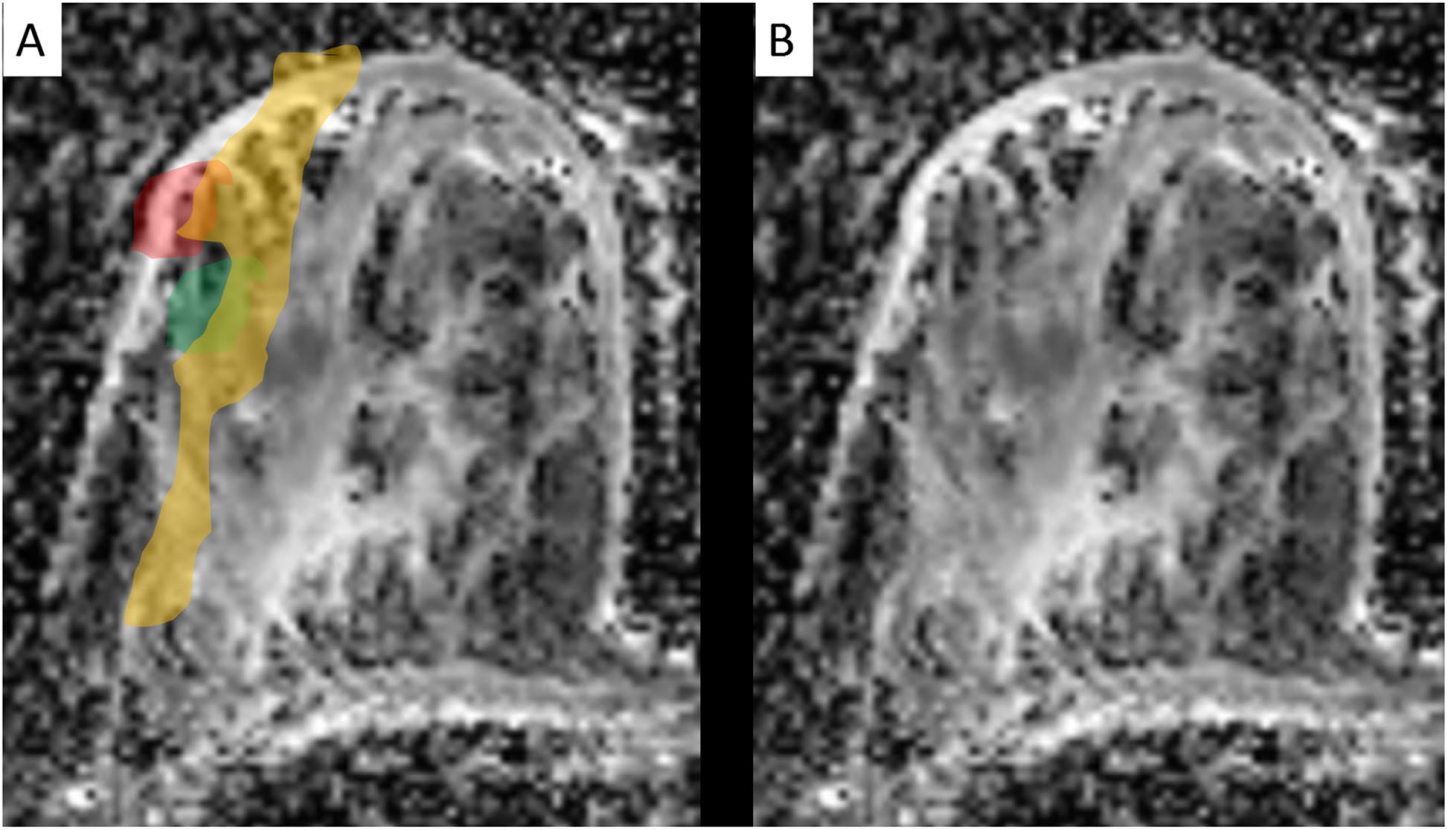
Apparent diffusion coefficient (ADC) mapping (detailed analysis of the DWI already shown in Fig. 1). ADC values are extracted using quantitative region-of-interest-based measurements by standardised methods. ADC is given with (A) and without (B) colour overlap. ADC was applied to distinguish ductal carcinoma *in situ* (DCIS, orange) from invasive carcinoma (red). Note the presence of a benign lesion (a fibroadenoma), also correctly characterised by the ADC map (green), adjacent to the DCIS
